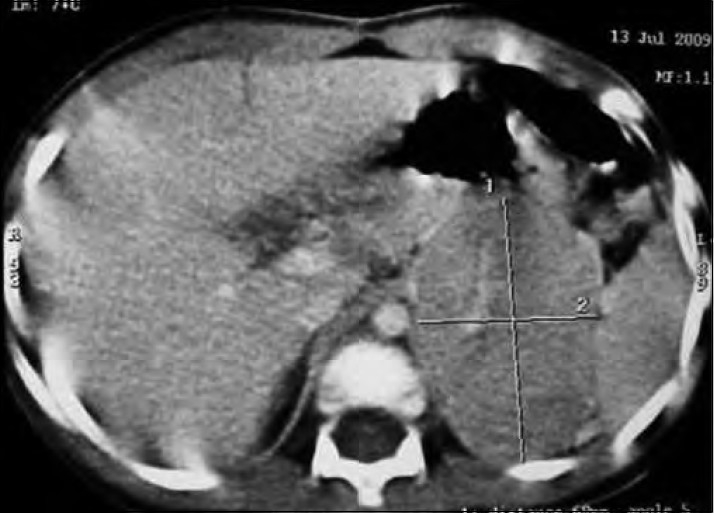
Contrast enhanced computed tomography-left suprarenal mass showing rim-like enhancing capsule
